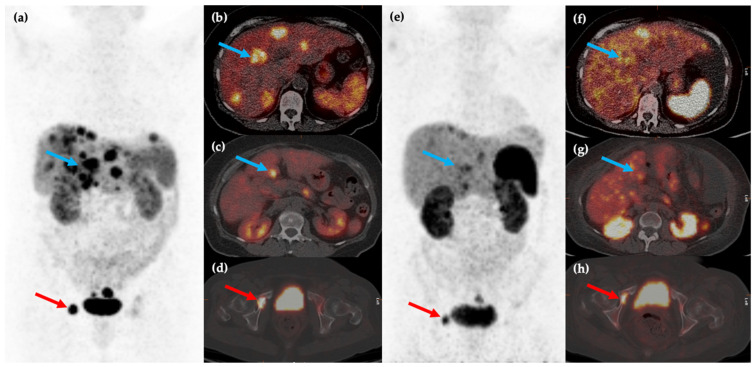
Fifty-year-old female with metastatic G1 NET to liver and bones. ^68^Ga-DOTATATE PET/CT MIP (a) and axial (b–d) images show multiple sites of SSTR positive hepatic (blue arrows) and osseous (red arrows) disease. The patient was treated with ^177^Lu-DOTATATE with favorable partial response on post-therapy ^68^Ga-DOTATATE PET/CT MIP (e) and axial (f–h) images, which show reduction in size and/or number of the previous sites of disease.
